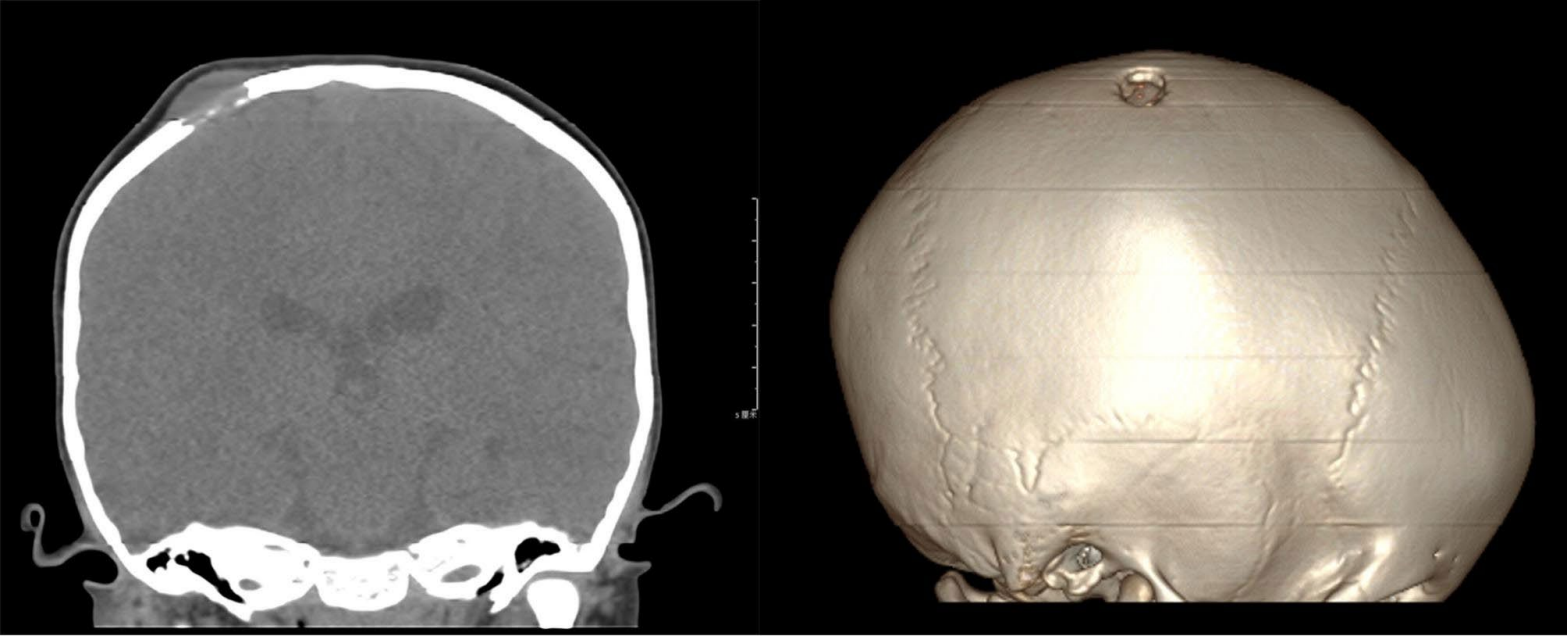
The LCH CT with cranial lesion, the CT showed bony destruction of the skull with chisel-like changes with ill-defined borders
